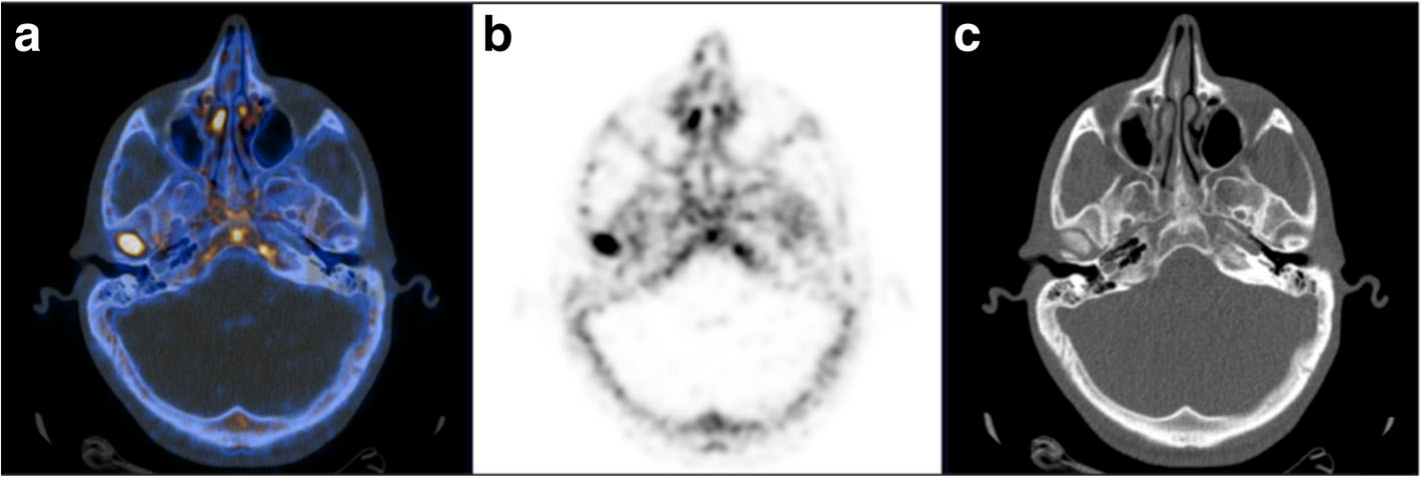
a axial 18F–fluoride PET/CT fusion scan of a patient affected by UCH in active growth: radiotracer uptake on right mandibular condyle; b axial 18F–fluoride PET scan only; c axial CT scan only
